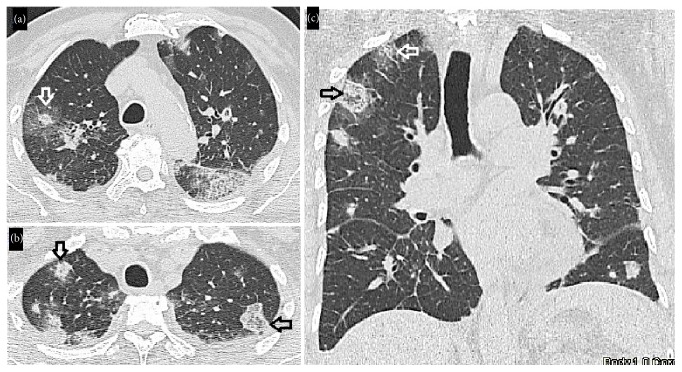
Chest CT scan. (a) Consolidations with peripheral ground-glass opacities compatible with halo sign in the apical segments of the upper lobes (white arrow). (b) Nodule with central ground-glass density and consolidation ring (reversed halo sign) in the apical segment of the upper lobes (black arrow). (c) Coronal reconstruction. Multiple peripheral nodules, some of them with reversed halo sign (black arrow) and halo sign (white arrow).
